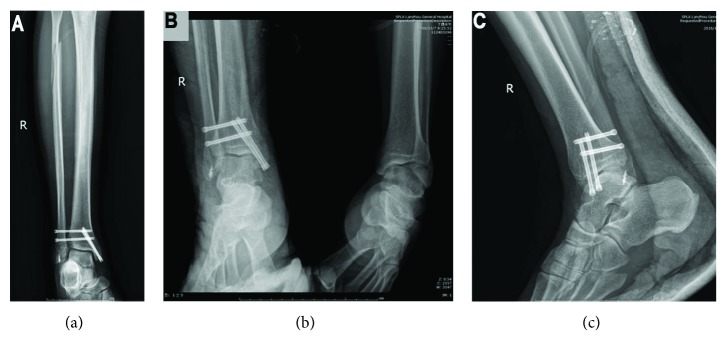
Posttreatment anteroposterior radiographs of the right shin and ankle and lateral radiograph of the right ankle. (a) Anteroposterior radiograph of the right shin and foot. (b) Anteroposterior radiograph of the right ankle. (c) Lateral radiograph of the right ankle.
